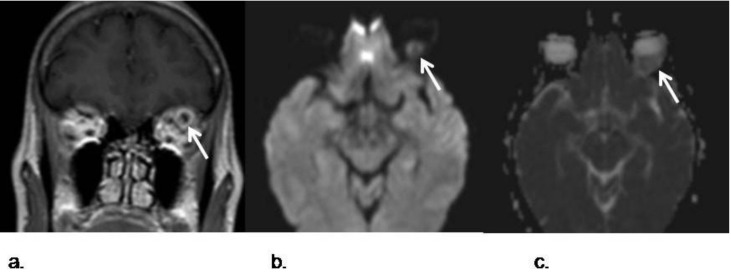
Intraorbitary metastasis of retroperitoneal leiomyosarcoma. (a) Postcontrast coronal TSE T1-weighted image of the orbits shows a left intraorbital nodule in the intraconal space. The lesions shows a ring-like pattern of enhancement with a central hypointense area. This appearance was not specific, as many masses in the orbit may have similar presentation, like an abscess or tumors; (b) DWI with a *b* value of 1,000 s/mm^2^; (c) corresponding ADC map show a marked restriction of the lesion that favours malignancy. Posterior surgical removal confirmed a metastasis from a retroperitoneal leiomyosarcoma.
